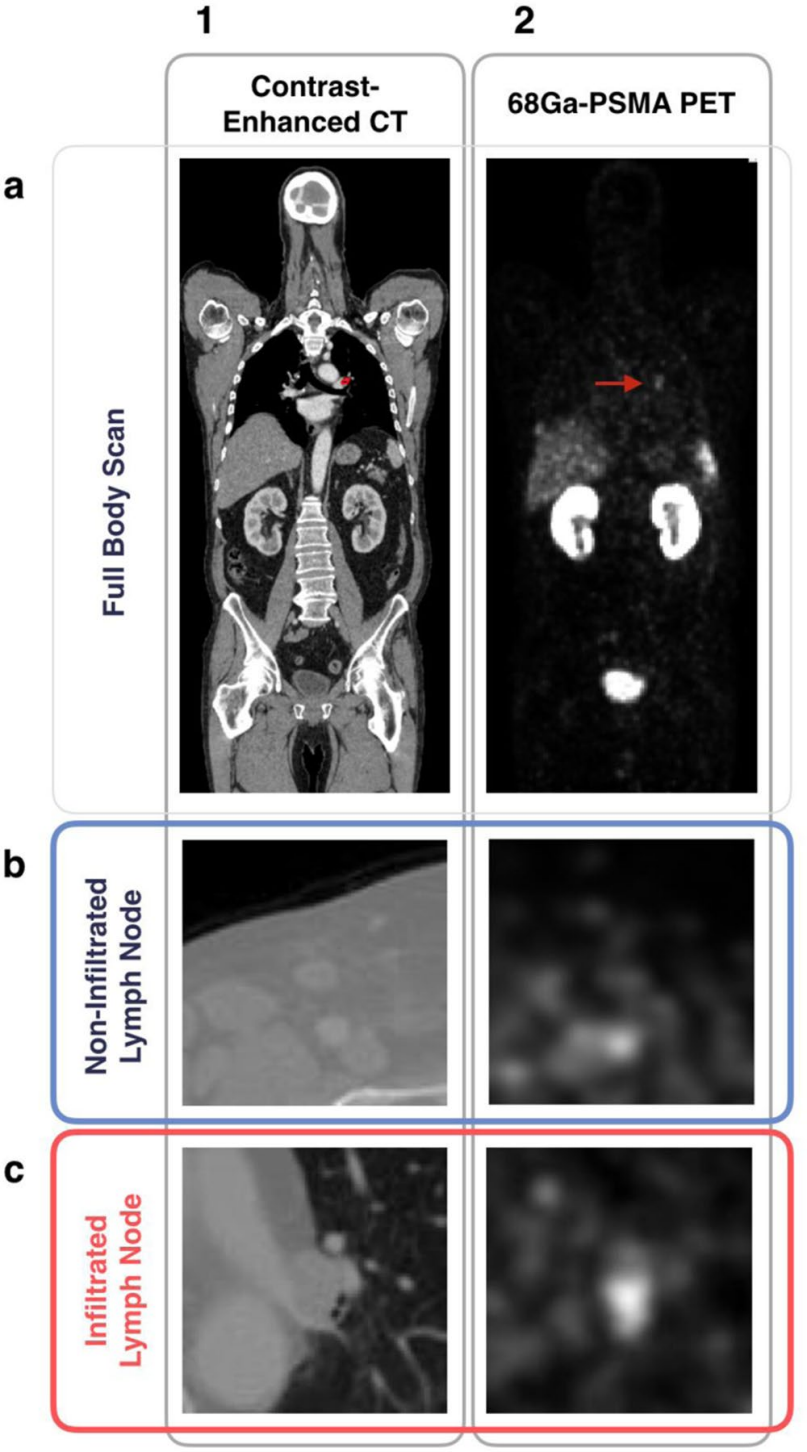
Generation of Labelled Dataset. (a) Imaging of a single patient with (1) a contrast-enhanced CT scan and (2) a 68Ga-PSMA PET scan. An average of 4.72 ± 0.77 lymph nodes were selected and semi-automatically segmented for each patient. A single lymph node positive for infiltration by PCa can be seen in the mediastinal region outlined in red in the CT image in (a1), and demarcated by a red arrow in PET scan in (a2). Using the 68Ga-PSMA PET/CT as our reference standard, a label for infiltration status by prostate cancer (either positive or negative) was assigned on a per lymph node basis. (b) An example of a negative 68Ga-PSMA PET/CT image pair in which the centered lymph node does not exceed background. (c) An example of a positive image pair.
